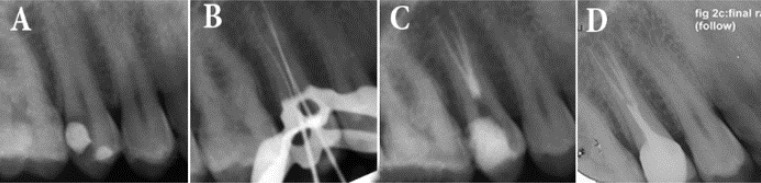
A)* Initial periapical radiography; *B)* Working radiography showing three files in three canals; *C)* Final radiography and CBCT; *D)* Follow-up radiography after six months
